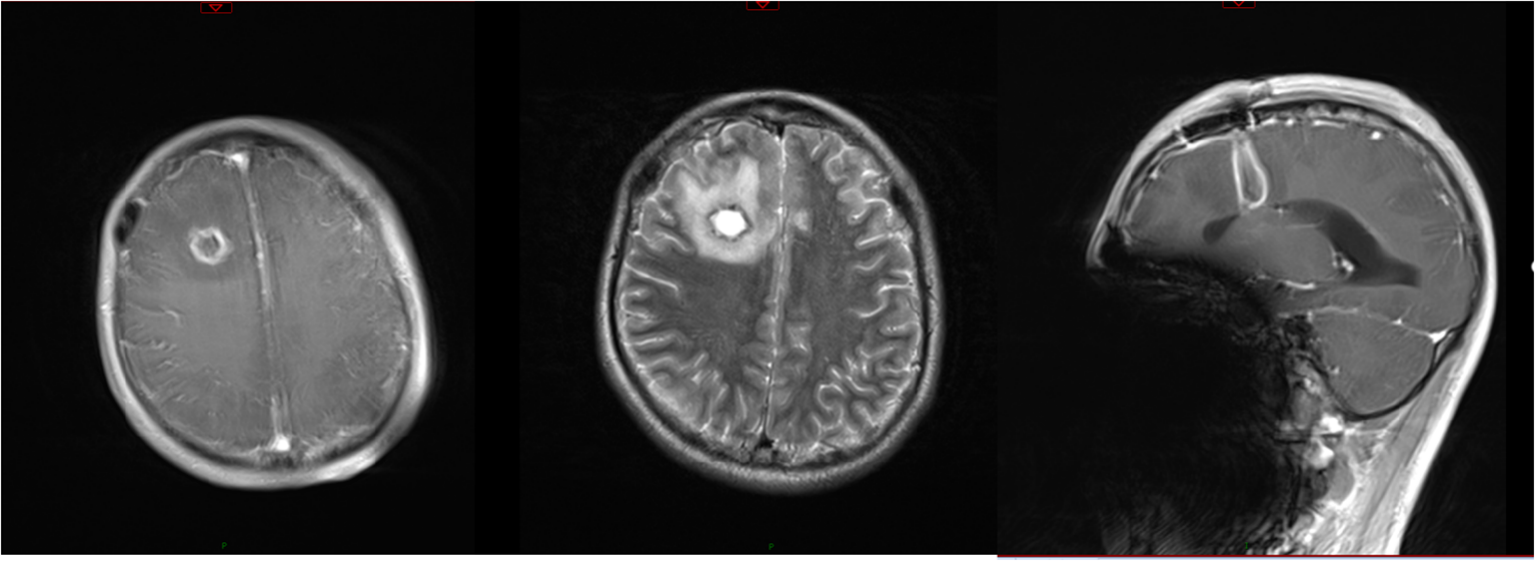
Results of MRI before the new therapeutic regimen. T2 scanning showed that the T2 signal from the interior of the lesion was long, while that from the surrounding cystic wall was short. Enhanced MRI scanning showed significant enhancement along the surgical path. The lesion localized in the surgical area formed a complete cystic wall and was significantly enhanced.
